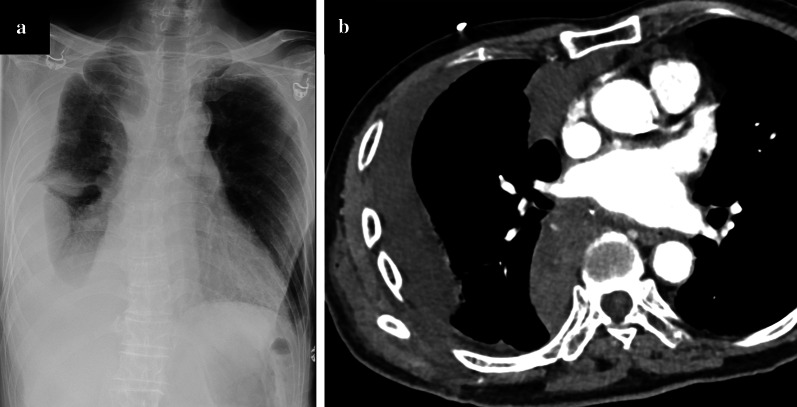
a Chest X-ray and b contrast-enhanced chest computed tomography of the patient showing a right haemothorax (performed in the emergency room)
